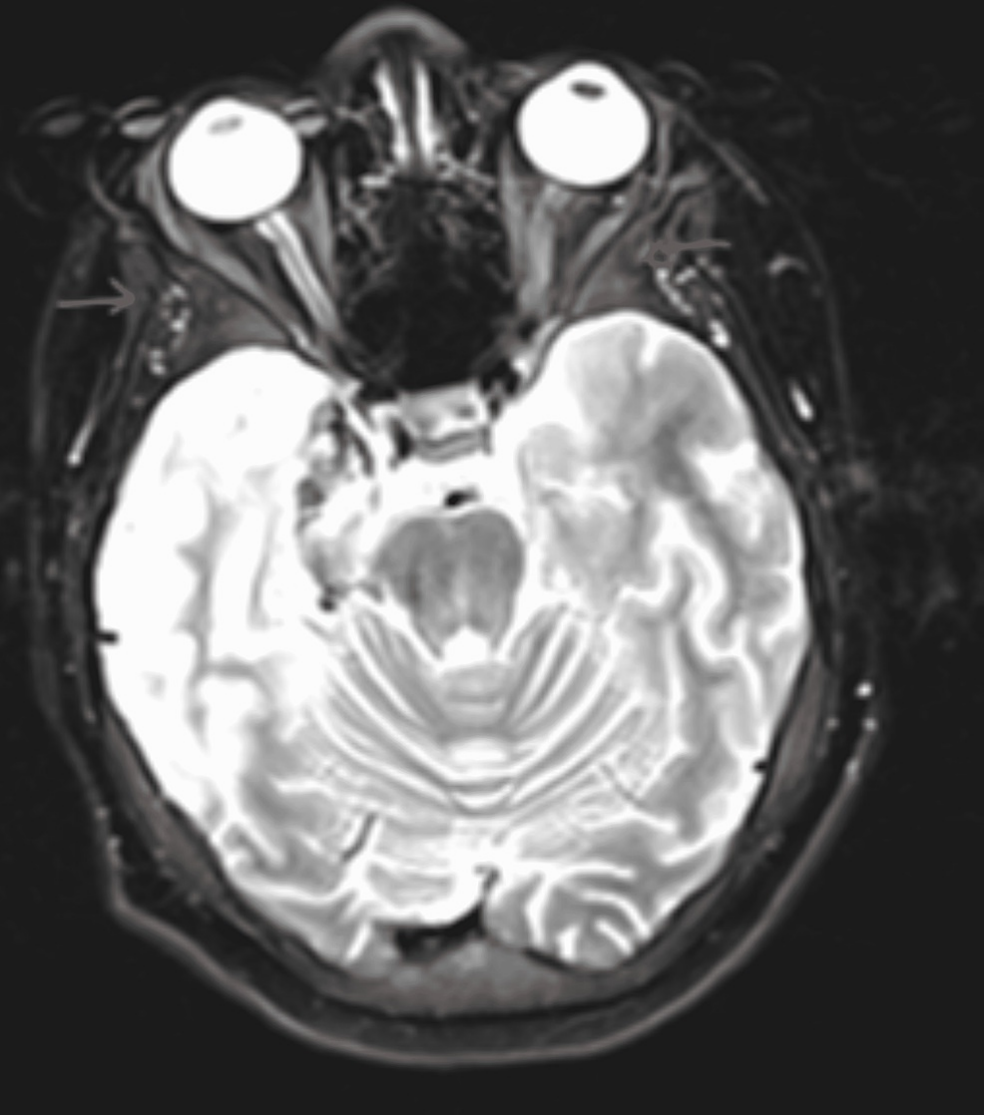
MRI brain with contrast focused on orbits, T2-weighted, axial view The axial view shows bilateral ocular globes and extra-ocular muscles that are normal.
